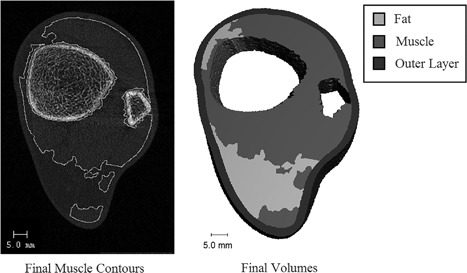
Representative HR‐pQCT scan at the distal tibia. Reprinted from Journal of Clinical Densitometry, vol. 20, MC Erlandson, AKO Wong, E Szabo, N Vilayphiou, MA Zulliger, JD Adachi, AM Cheung, Muscle and myotendinous tissue properties at the distal tibia as assessed by high‐resolution peripheral quantitative computed tomography, pages 226–232, Copyright (2016), with permission from Elsevier.
